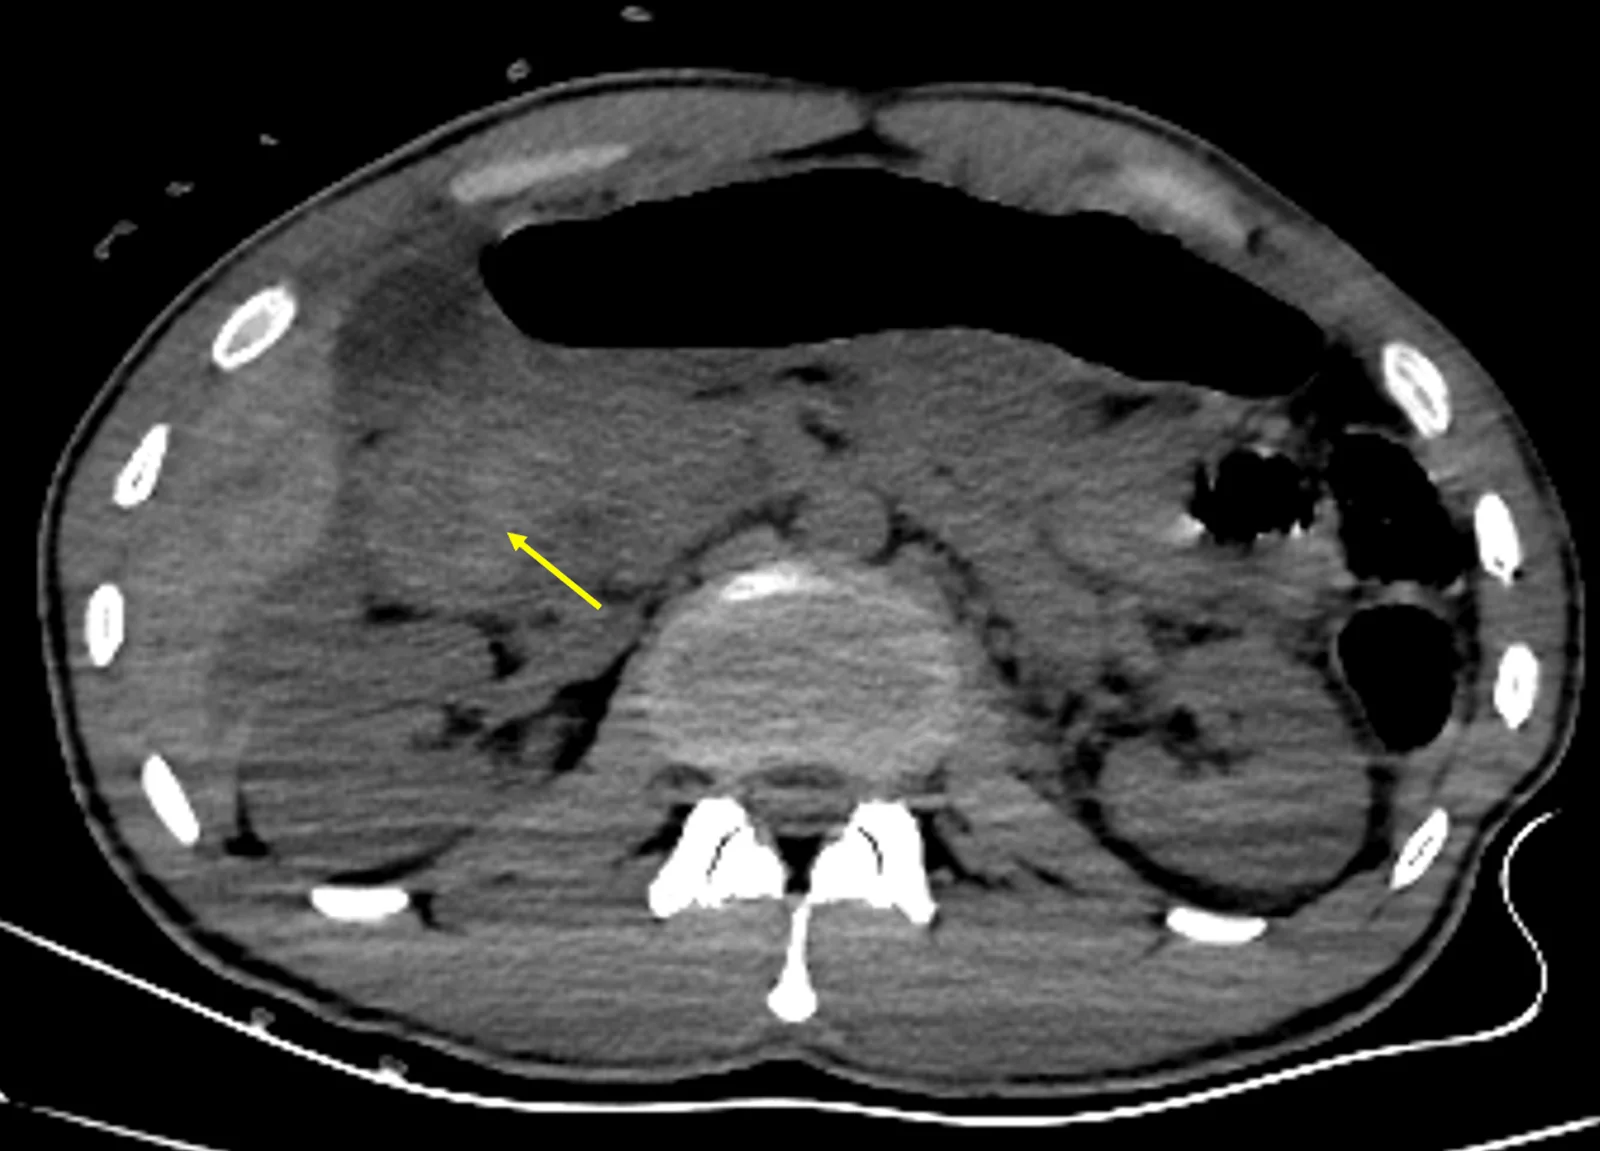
Imaging findings from abdominal non-contrast CT Axial abdominal CT without contrast shows focal spontaneous hyperdensity of the duodenal wall at D1 (yellow arrow).
